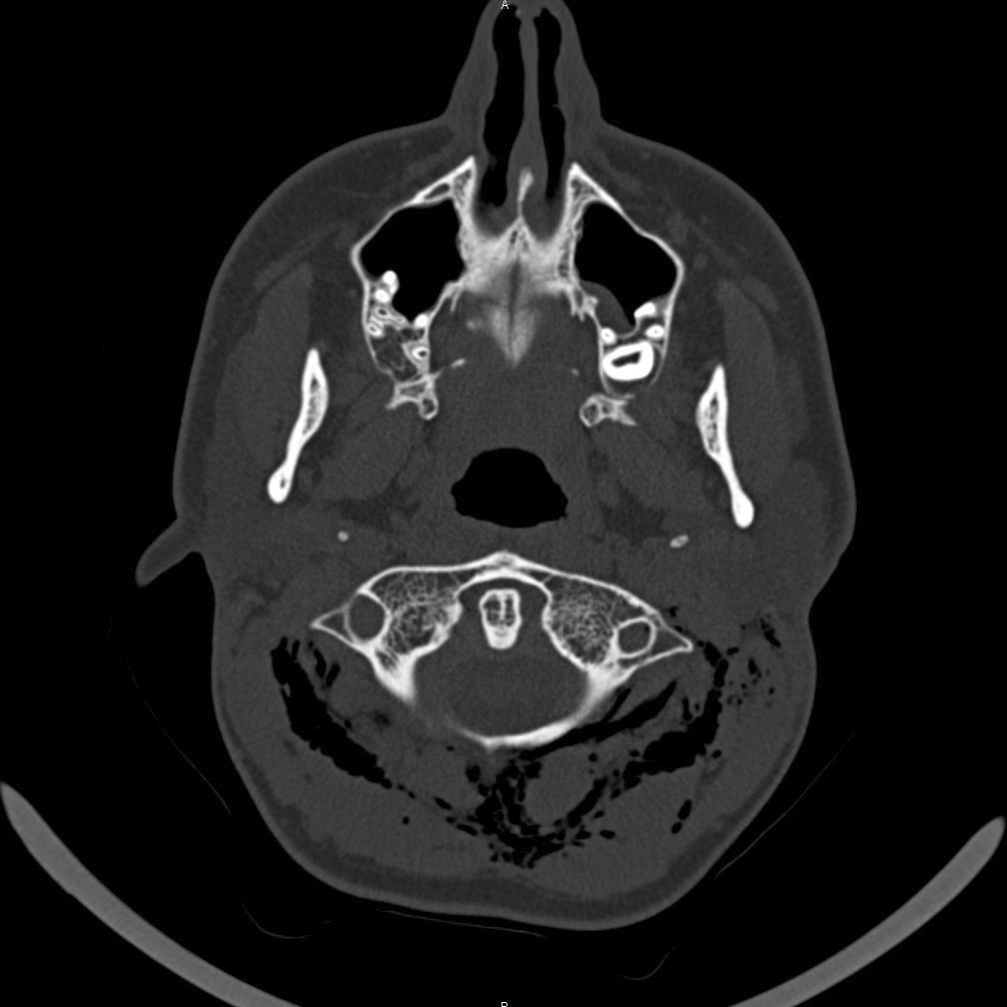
Axial plane HRCT scan – bilateral SCE evident at the level of C1‐C2, between the suboccipital muscles bilateraly
